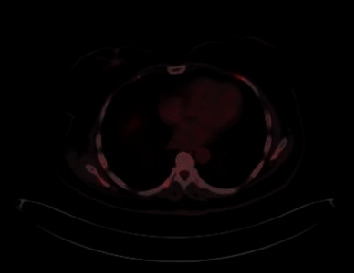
Whole body PET/CT showing right breast suspicious mass.
